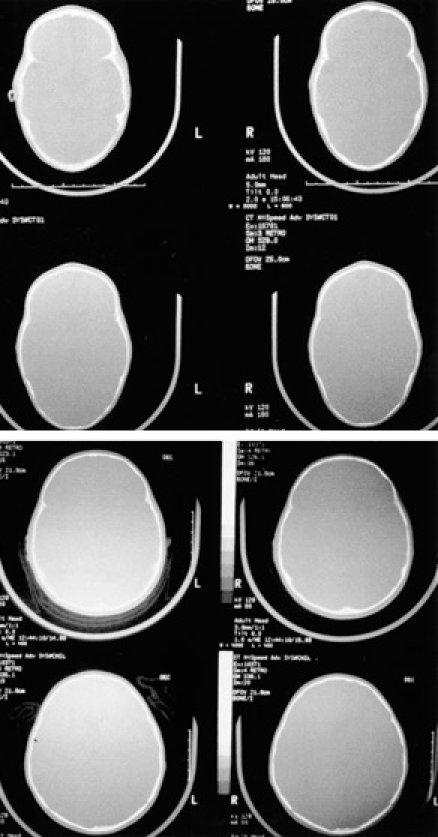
Fig. 13 a, b. 3D CT scan in scaphocephaly a – Preoperatory; b – Postoperatory
